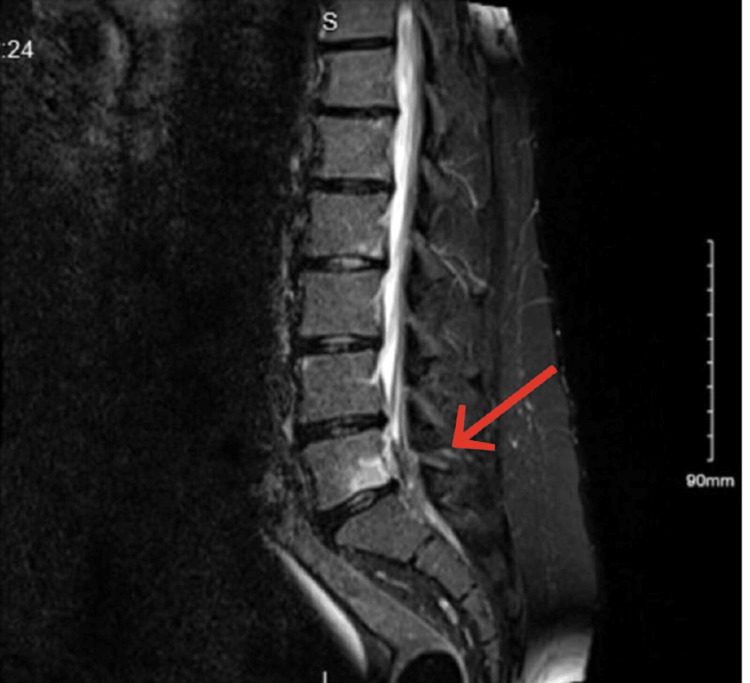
MRI revealed a paracentral left disc herniation at the L5-S1 level, along with Modic type 1 changes on the inferior endplate of the L5 body.
